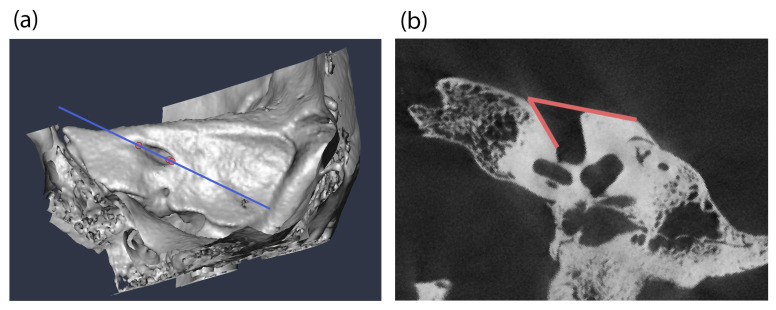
Overview of the lateral angle measurement procedure. (a) The intersection of the internal acoustic meatus in the midsagittal plane using the landmark-based method on CT scans. Landmarks are depicted as red dots. (b) Measurement of the lateral angle in the CT scan slide aligned to the midsagittal plane of the internal acoustic meatus.
